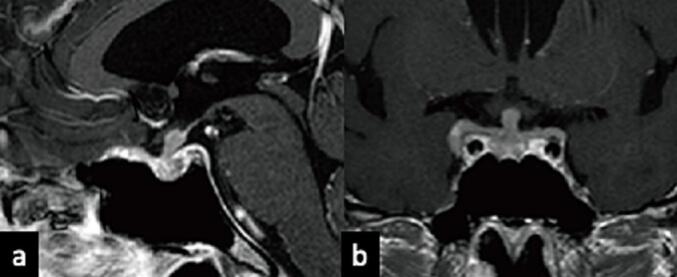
Preoperative T1-weighted image with contrast after steroid administration showing no tumor shrinkage (a: sagittal, b: coronal).
